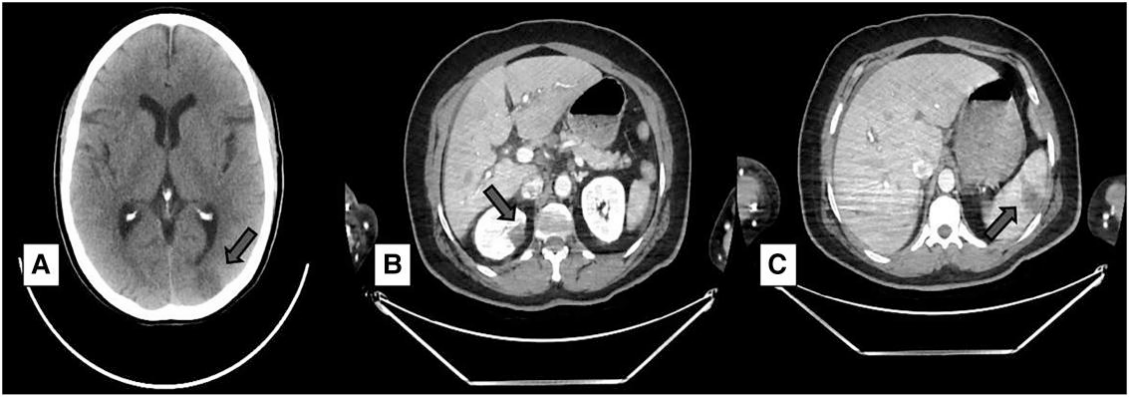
Computed tomography angiography showing embolism in the brain (*A*), kidney (*B*), and spleen (*C*).
